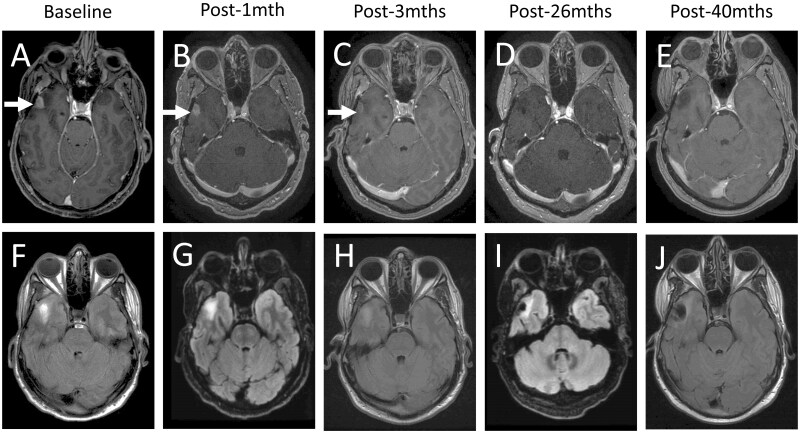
Brain MRIs showing durable complete response for patient. Patient with recurrent right temporal glioblastoma failing chemoradiation with 7 cycles of adjuvant temozolomide with Optune^®^ device, then re-resected gross total resection confirmed active glioblastoma, and subsequently progressed after 2 cycles of lomustine before starting pemigatinib on study. A-E: axial post-gadolinium MRI T1 sequences showing resolution of enhancing temporal mass over time; F-J: axial MRI T2/FLAIR sequences showing the resolution of edema over time.
